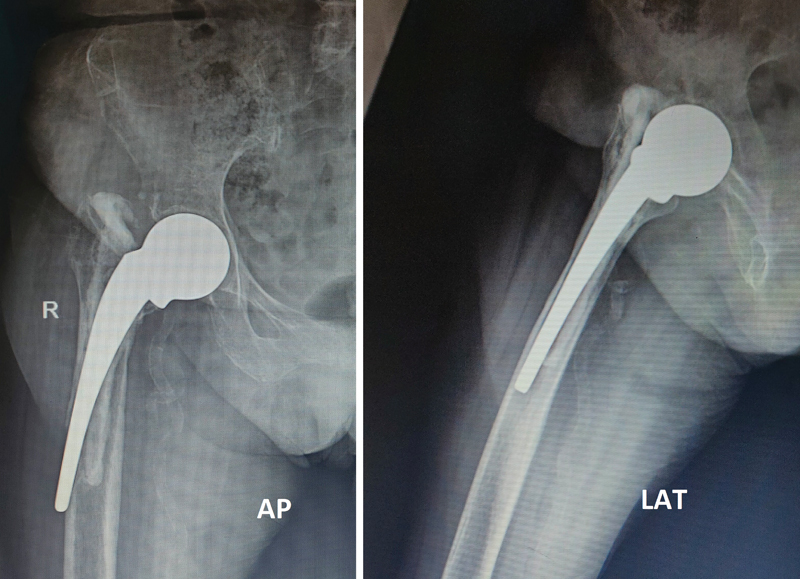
Preoperative radiograph on anteroposterior (AP) and lateral views.
